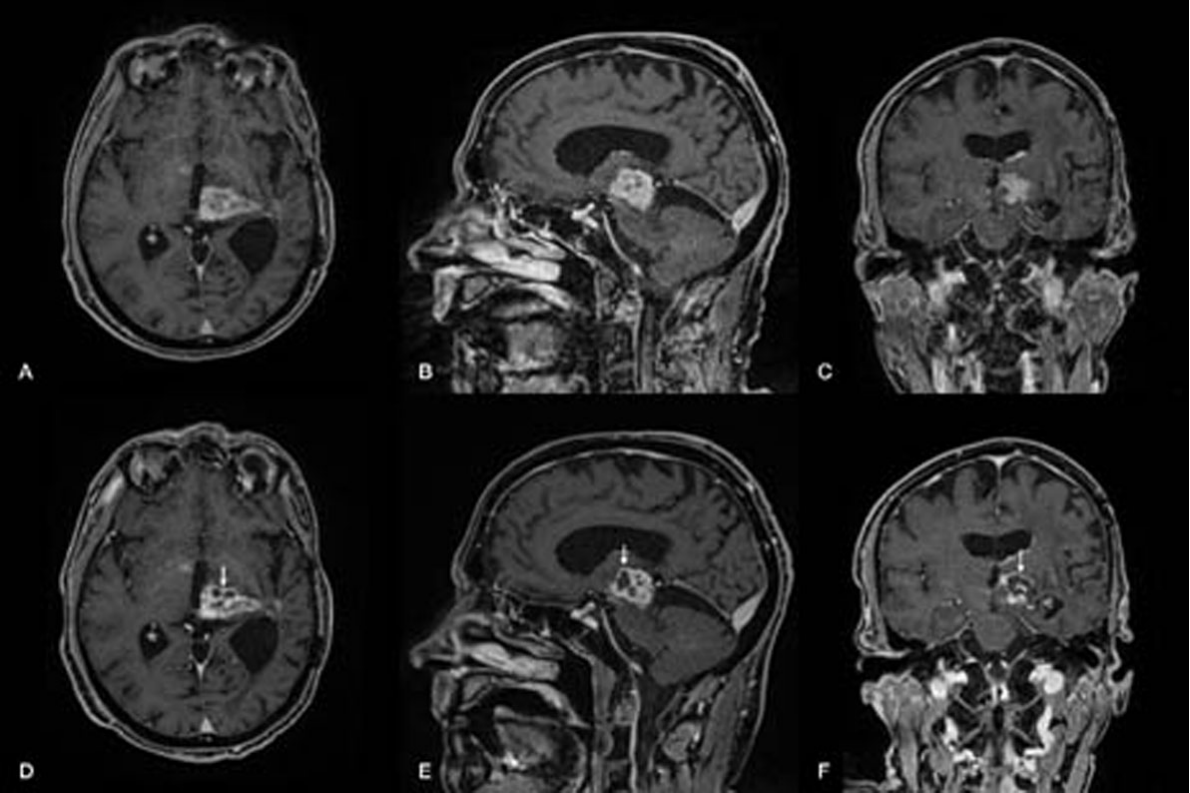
# First non-invasive thermal ablation of a brain tumor with MR guided focused ultrasound
